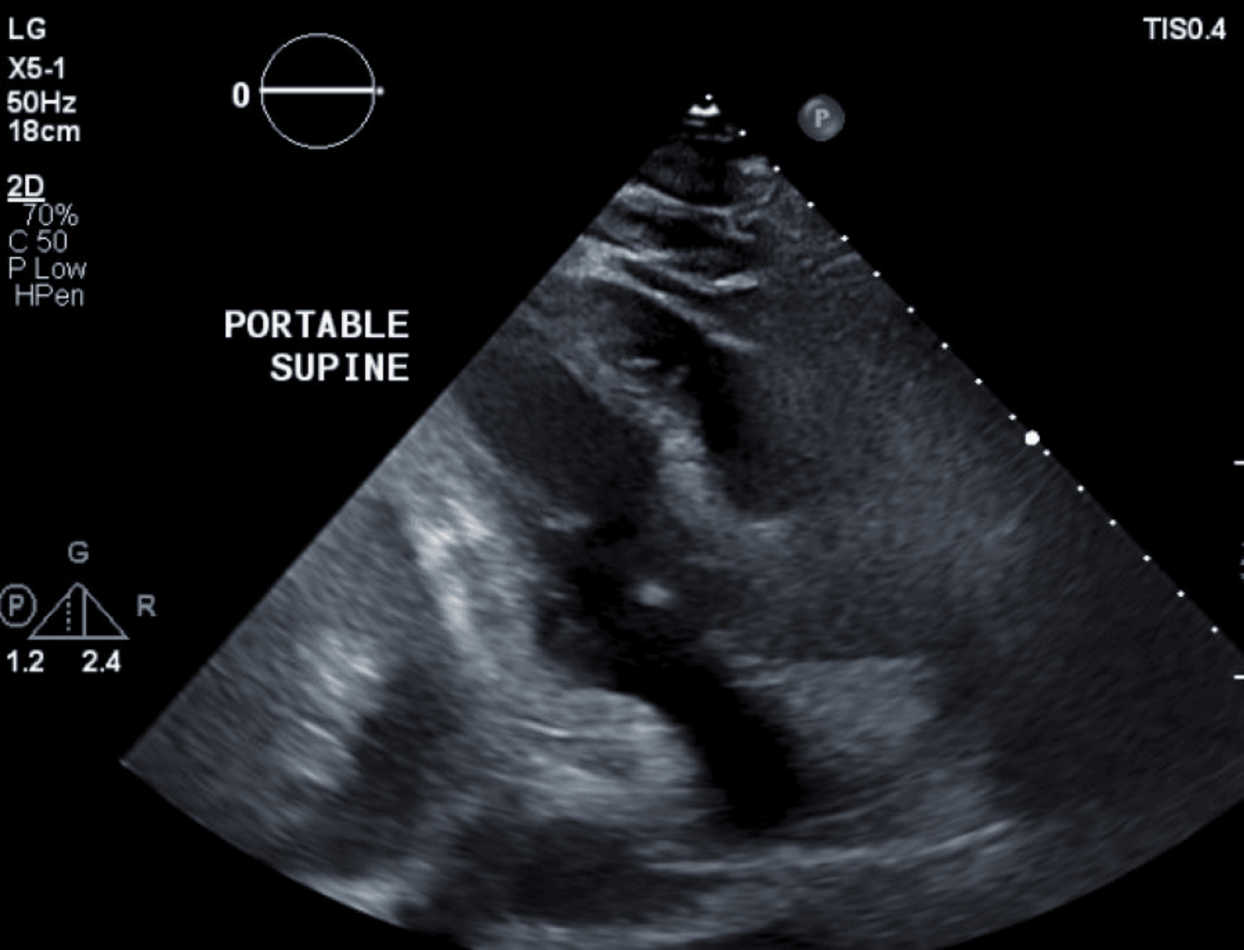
Transthoracic echocardiography showing normal left ventricular systolic function (ejection fraction (EF) 65%–70%) with mild (grade I) diastolic dysfunction
